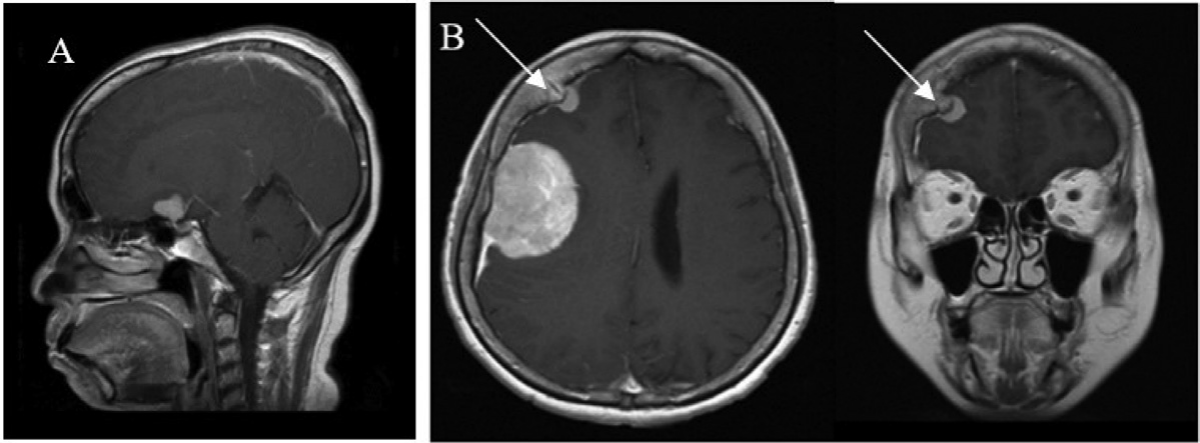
MRI meningiomatosis. (A) T1+ gadolinium contrast sagittal section showing mass in the suprasellar. (B) T1+ gadolinium contrast axial and coronal section showing hyperostosis of os frontalis dextra (white arrow).
